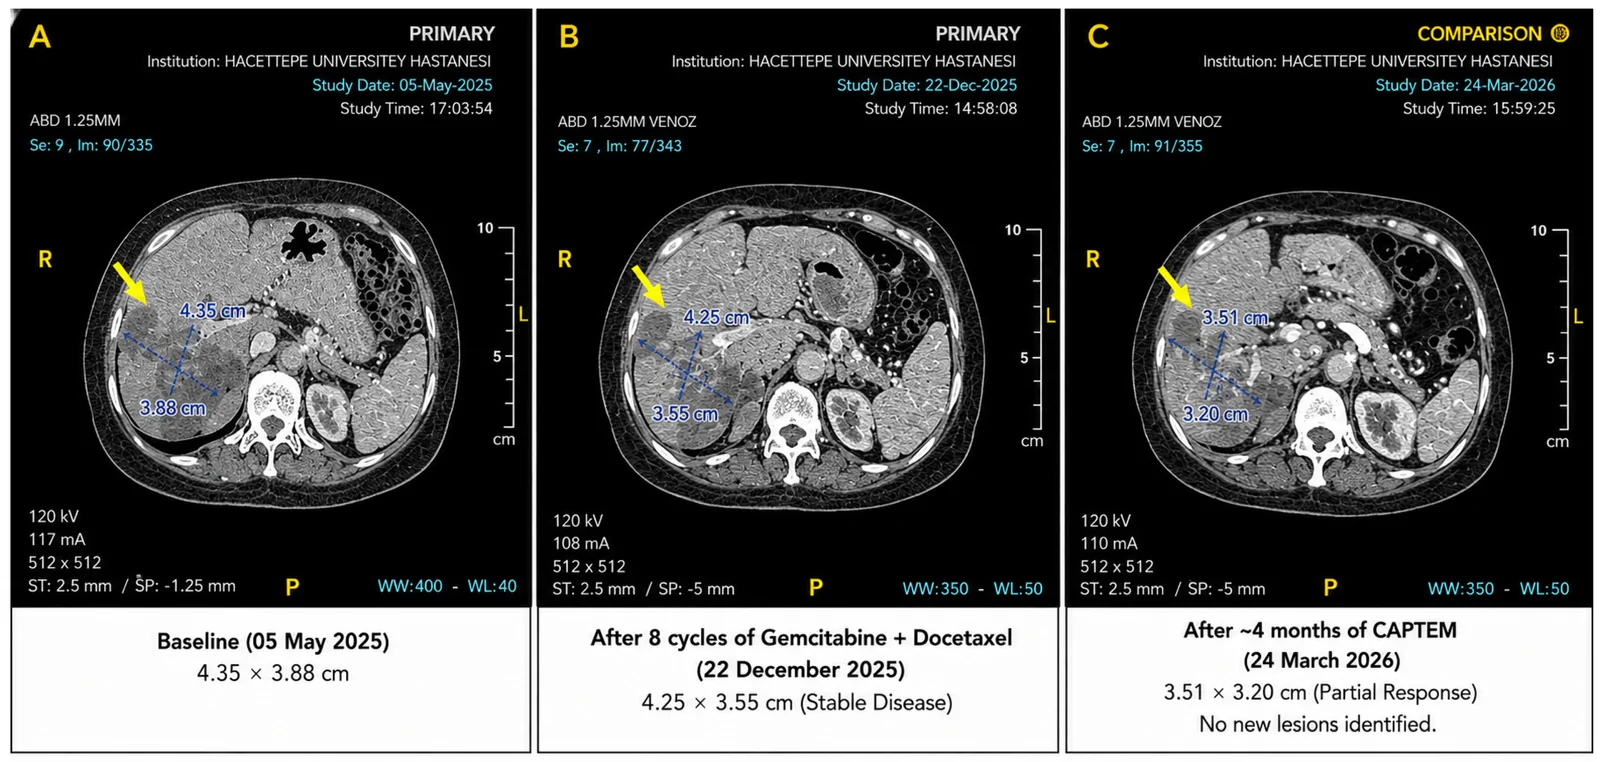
Serial contrast-enhanced CT images obtained in the venous phase demonstrating the treatment response of the same target hepatic lesion (arrows) at comparable anatomical levels. (A) Baseline CT (5 May 2025) image showing the target hepatic metastasis measuring 4.35 × 3.88 cm. (B) CT image obtained after eight cycles of gemcitabine plus docetaxel (22 December 2025), showing an essentially unchanged lesion measuring 4.25 × 3.55 cm, consistent with stable disease. (C) CT image obtained after approximately four months of CAPTEM therapy (24 March 2026), demonstrating a reduction in the target lesion to 3.51 × 3.20 cm. No new lesions were identified.
